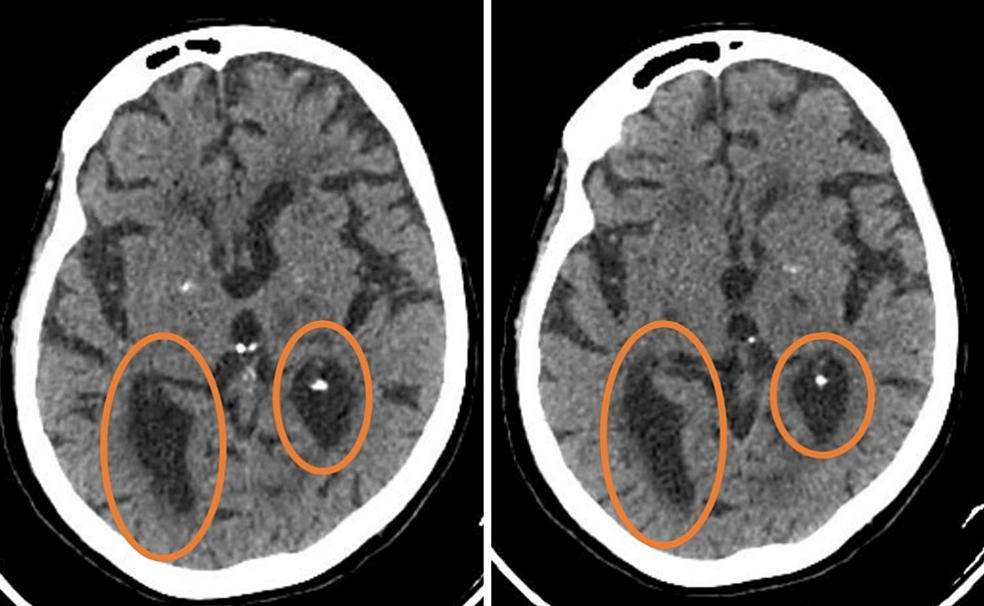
A non-contrast CT Scan of the head The marked circles show basal ganglia calcification.
